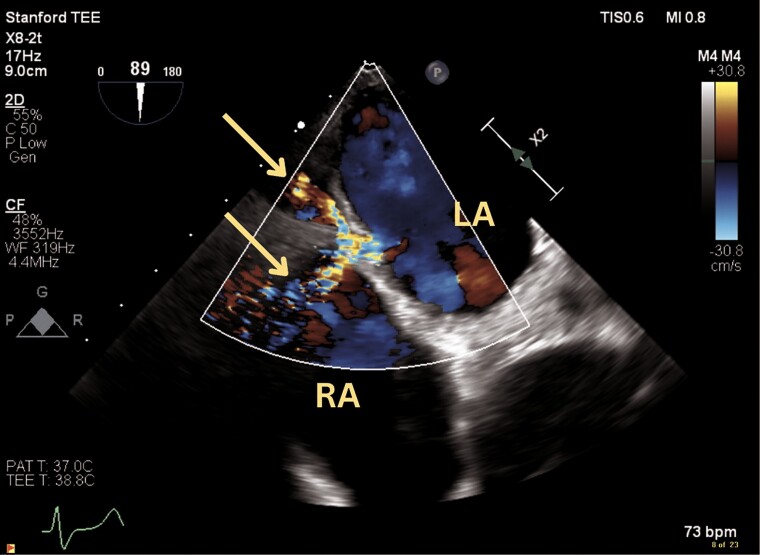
Modified bi-caval transoesophageal echocardiogram view with colour Doppler demonstrating flow from the left atrium into the interatrial septum dissection, pericardial space and right atrium (arrow). LA, left atrium; RA, right atrium.
